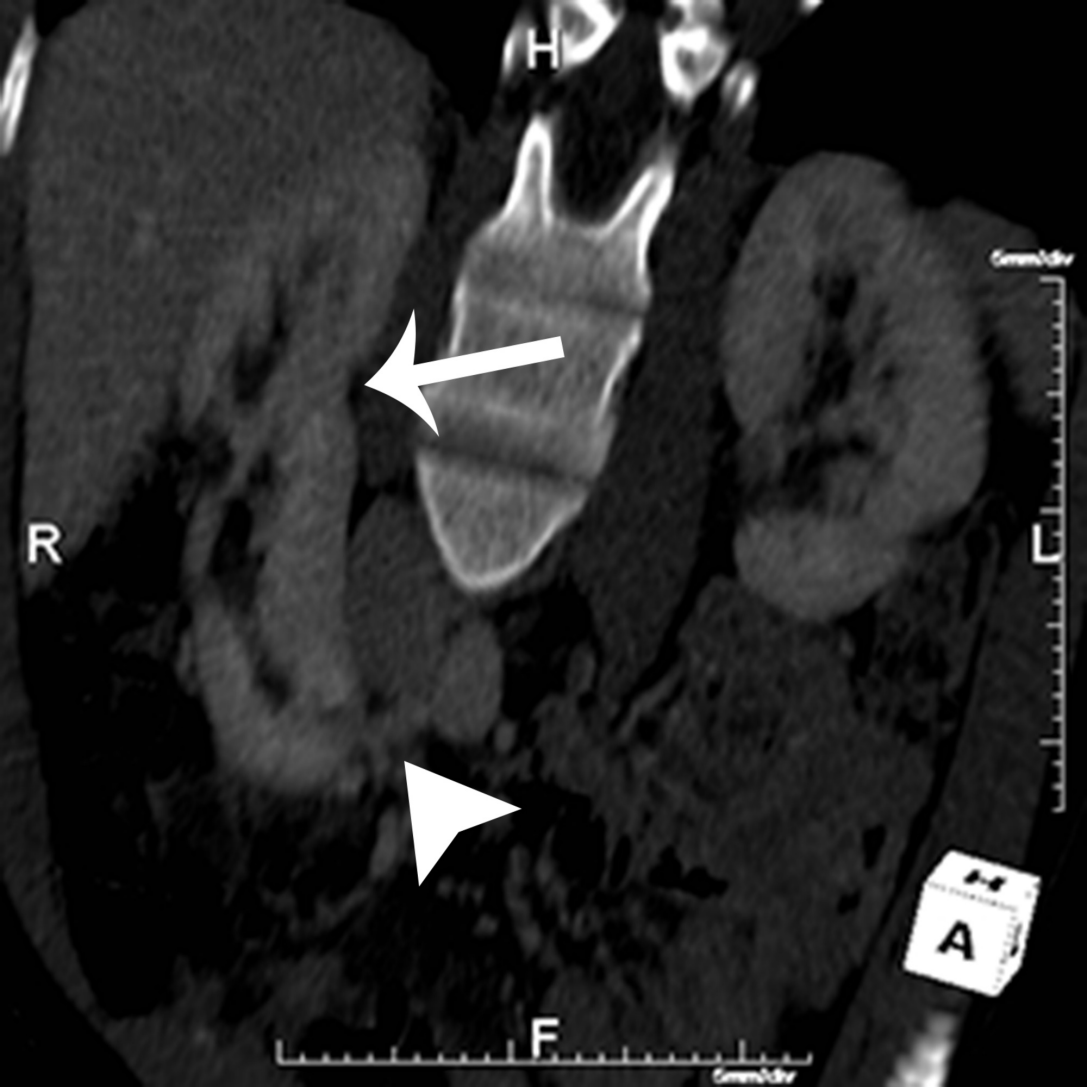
Coronal Oblique CT Reconstruction Coronal oblique reconstructed computed tomography (CT) image of the kidneys demonstrates bilateral native kidneys, as well as an ectopic supernumerary kidney fused to the lower pole of the native right kidney (white arrows). Additionally, the supernumerary kidney renal vein can be seen draining into the inferior vena cava (white arrowhead).
